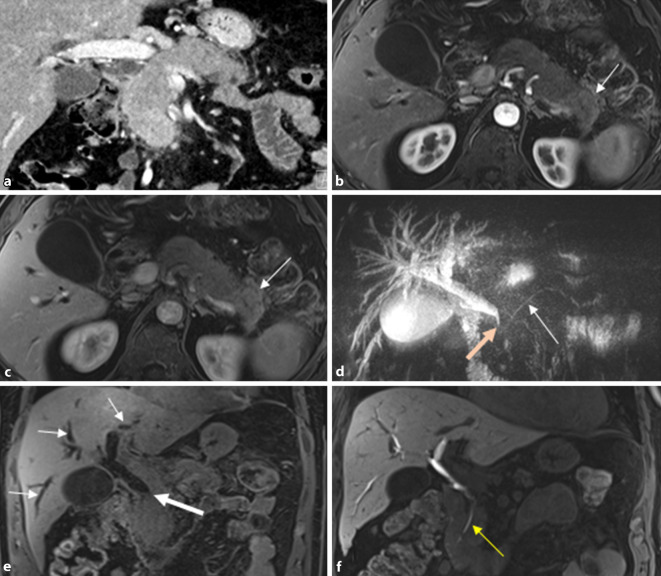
Die ausgeprägte Dilatation des Pankreashauptgangs sowie auch die Organatrophie fehlen bei der AIP typischerweise. Eine markante Gangdilatation oder abrupte Lumeneinengung weisen auf eine maligne Obstruktion hin, wohingegen langstreckige Einengungen des Hauptgangs oder multiple, segmentale Stenosen für eine fokale Entzündungsreaktion sprechen ([13, 17]; Abb. 5). In schwierigen Fällen können die diffusionsgewichteten Sequenzen (DWI) der MRT helfen, die AIP mit signifikant höheren ADC-Werten (Tab. 1) im Pseudotumor von einem Pankreaskarzinom zu unterscheiden [28].
